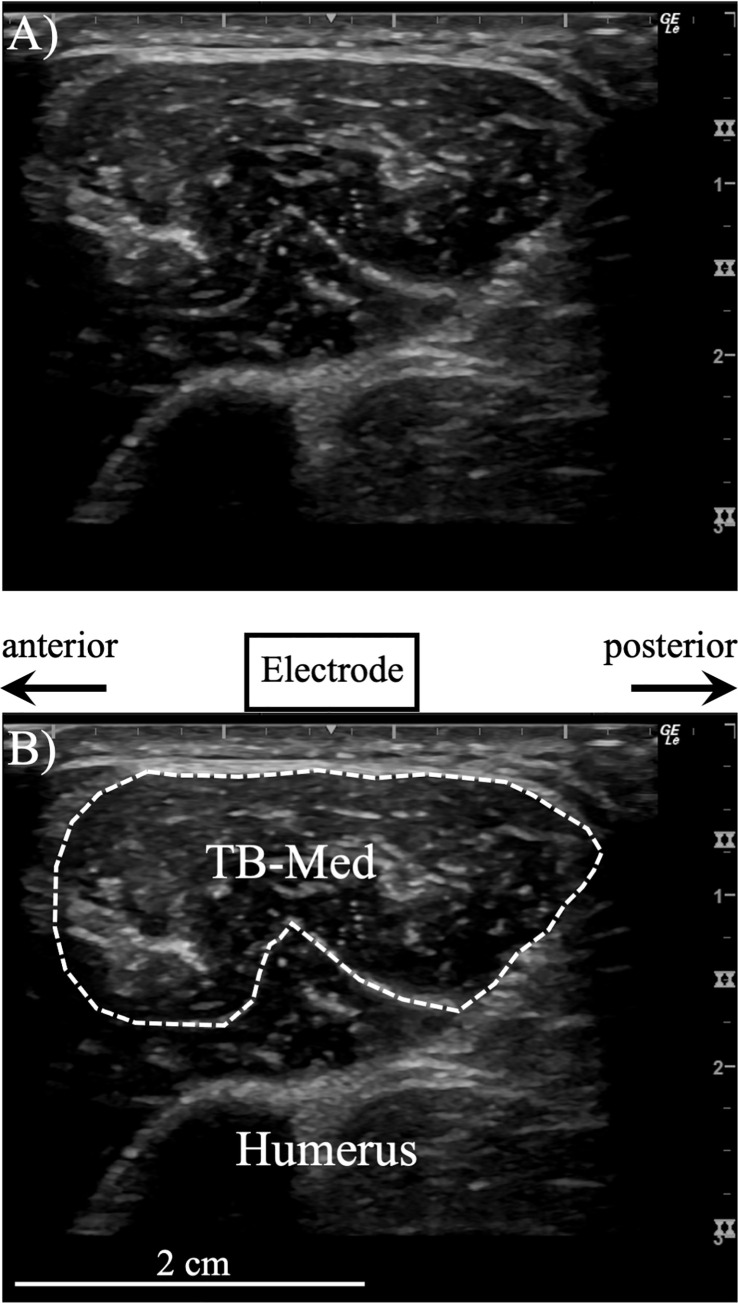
Representative B-mode ultrasound images. A) The original image. B) An image with information regarding the medial head of the triceps brachii (TB-Med) of a male subject. The electrode is 1 cm long and 0.5 cm high, and the long axis length of the TB-Med muscle is 3.1 cm.
